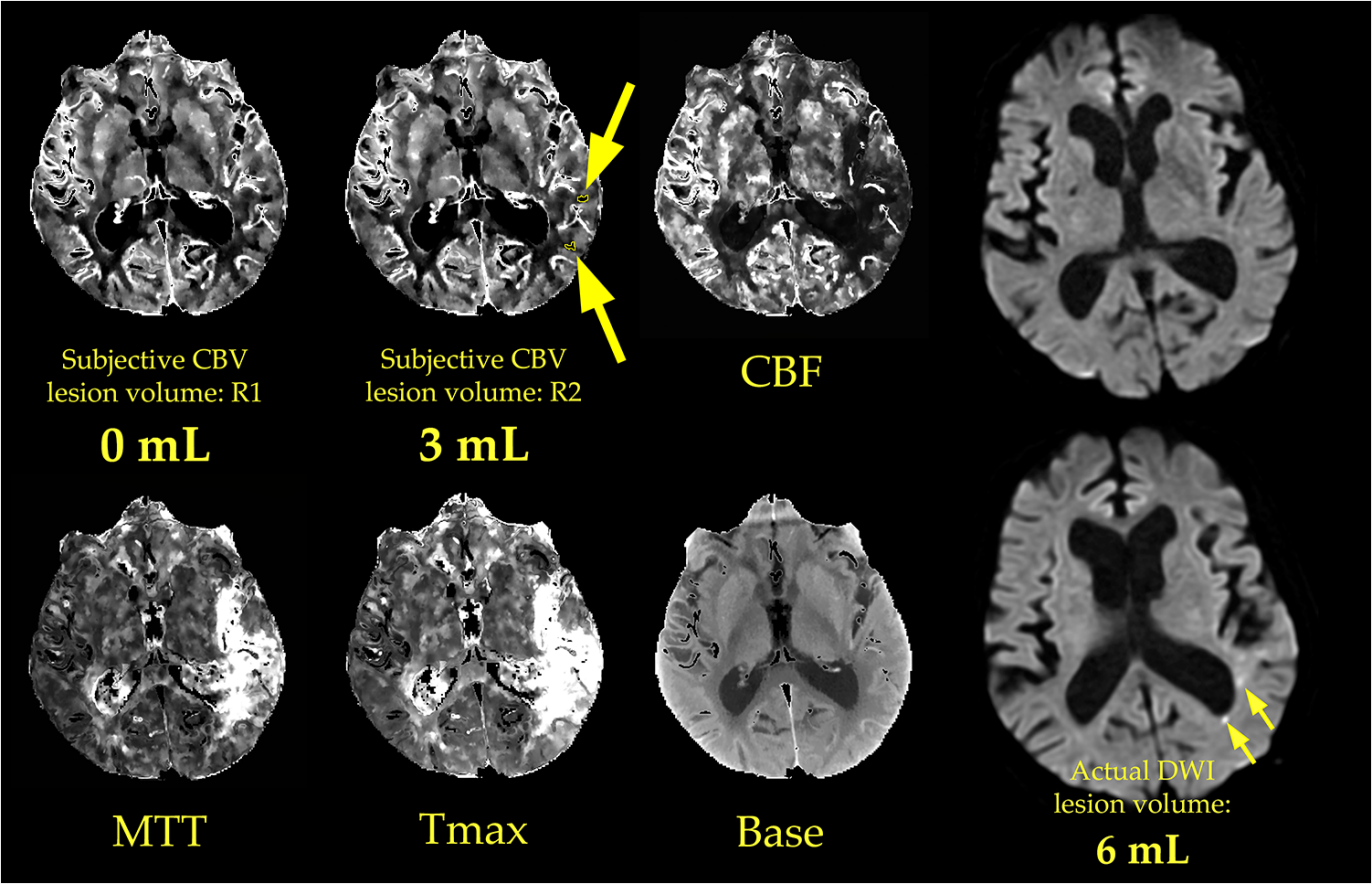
Subjective measurement of CBV lesion volume in a second patient. Two radiologists (R1 and R2) subjectively outlined regions of abnormally low CBV. In doing so, the radiologists had access to all available CTP images, and to clinical history (in this case, “aphasia and right face, arm, and leg weakness”). The patient and CT slice location are the same as those in Fig 3. R1 did not detect a lesion, i.e. he measured the core lesion volume to be 0 mL. R2 outlined several lesions (yellow outlines and large arrows) whose total volume was 3 mL. Although R2’s subjective lesion volume was very close to the actual DWI lesion volume of 6 mL, none of the CBV lesions that R2 outlined corresponded to actual DWI lesions. No lesions were present in a DWI image obtained at approximately the same level as these CTP maps (upper right), and all of the DWI lesions that were present were located more superiorly (lower right, small arrows).
